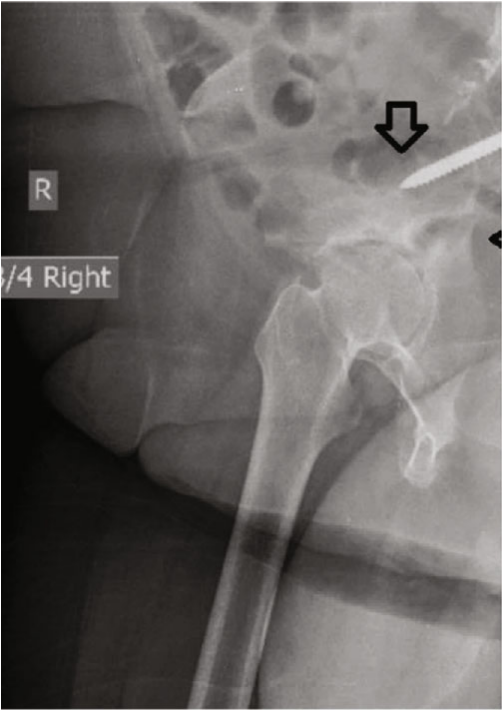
Right oblique Judet roentgenogram 18 months postoperatively showing the transverse fracture of the right acetabulum (long arrow) with disruption of the posterior acetabular column and the tip of the iliac screw (short arrow).
